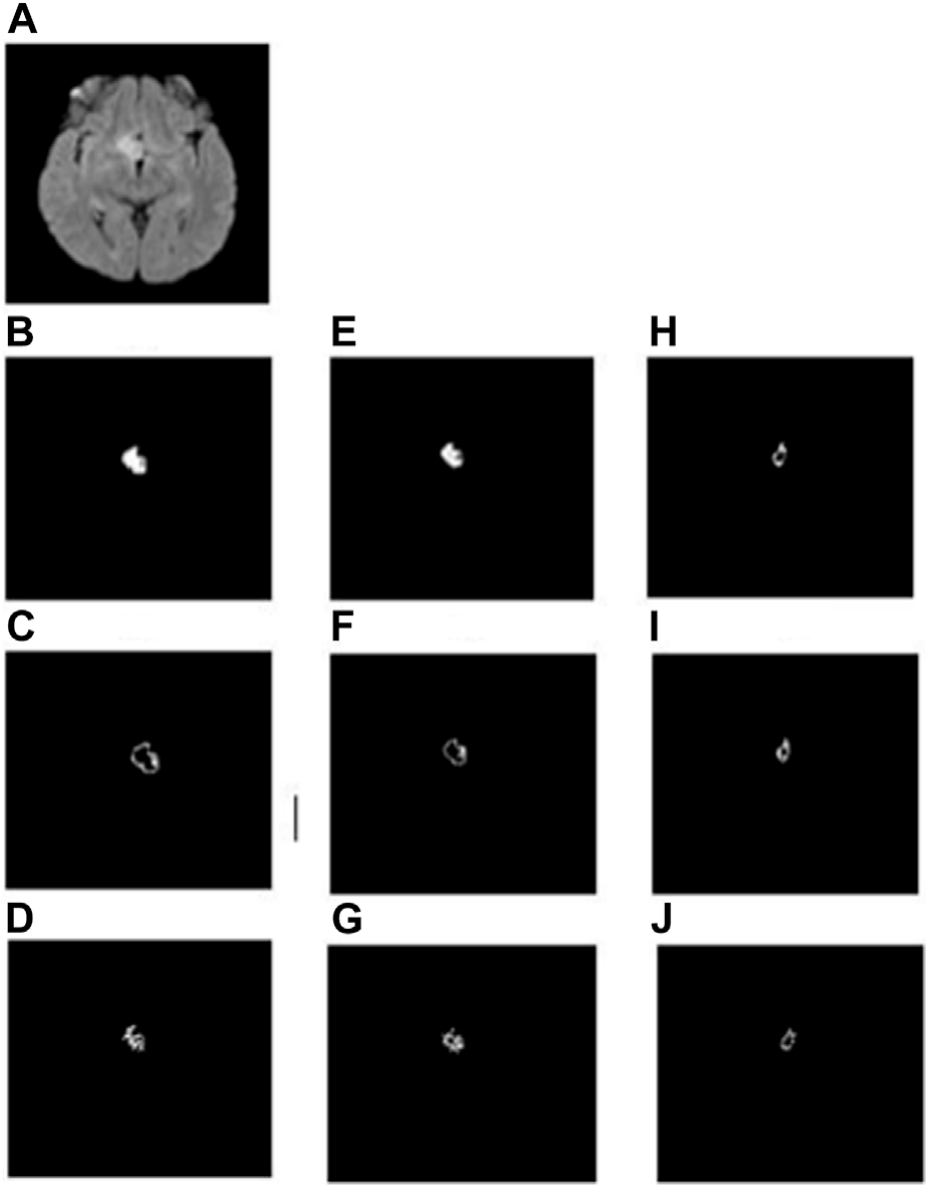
(A) FLAIR image of a typical LGG patient, (B–D) general structure, boundary, and skeleton of the whole tumor region, (E–G) general structure, boundary, and skeleton of the edema region, and (H–J) general structure, boundary, and skeleton of the enhanced tumor region, respectively.
